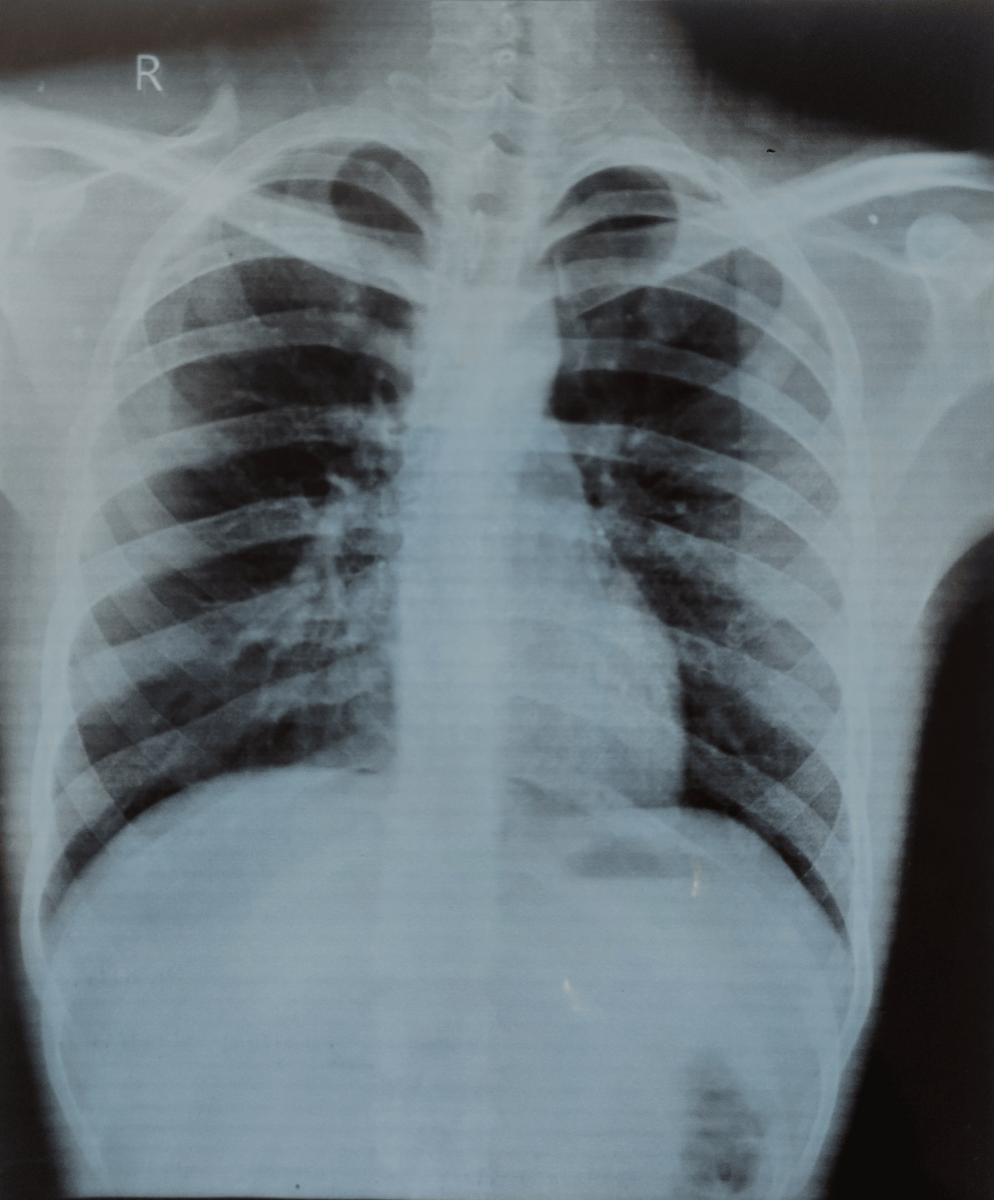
Post-treatment X-ray scan
